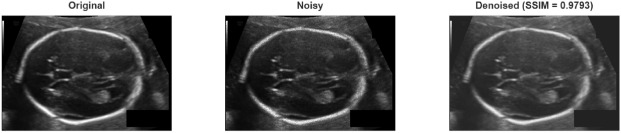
A sample result from fetal ultrasound dataset with Speckle noise.
